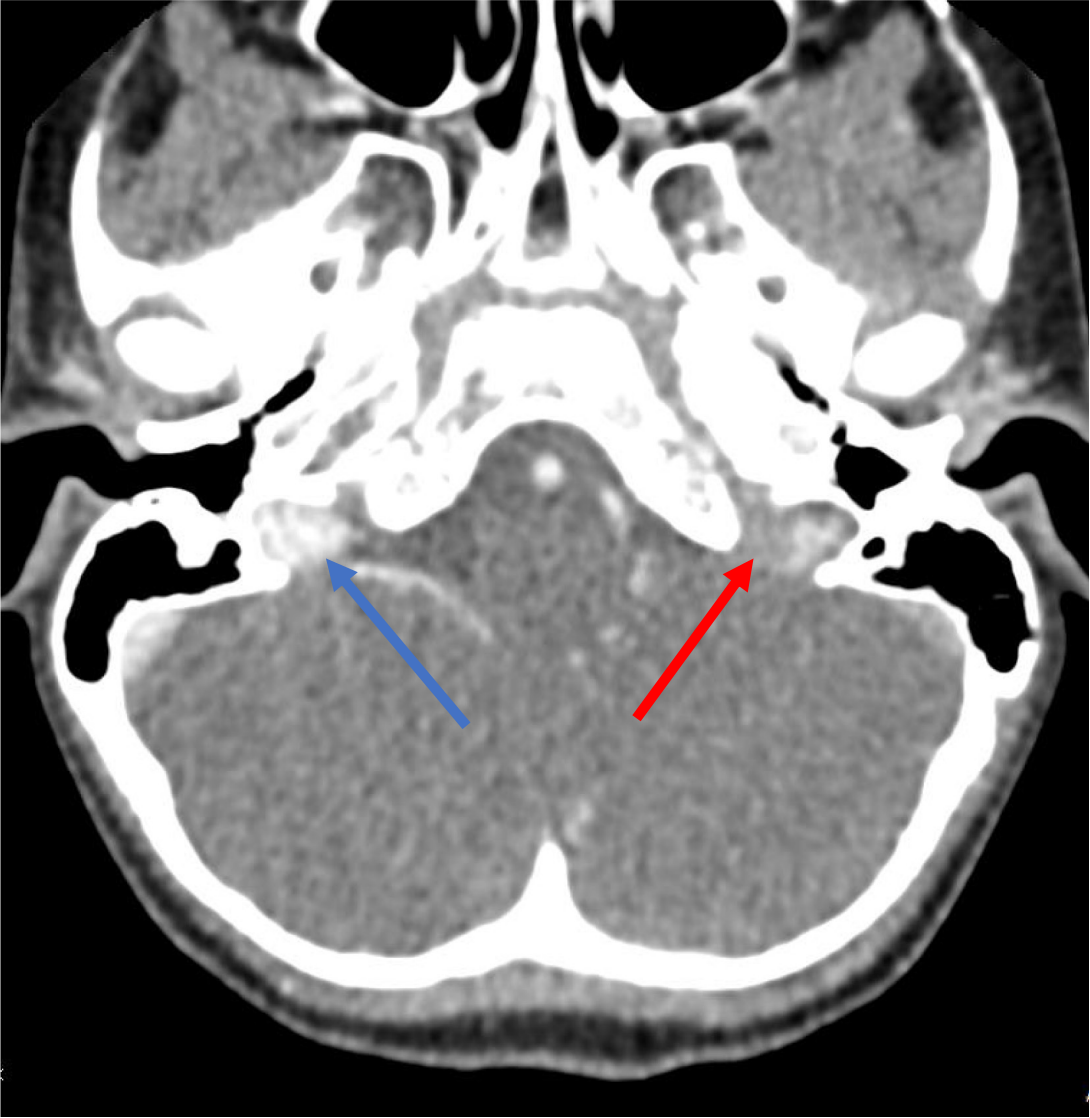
Axial contrast‐enhanced CT of the neck demonstrating normal contrast enhancement at the level of the right jugular bulb (blue arrow). Note the lack of contrast enhancement of the left jugular bulb (red arrow).
